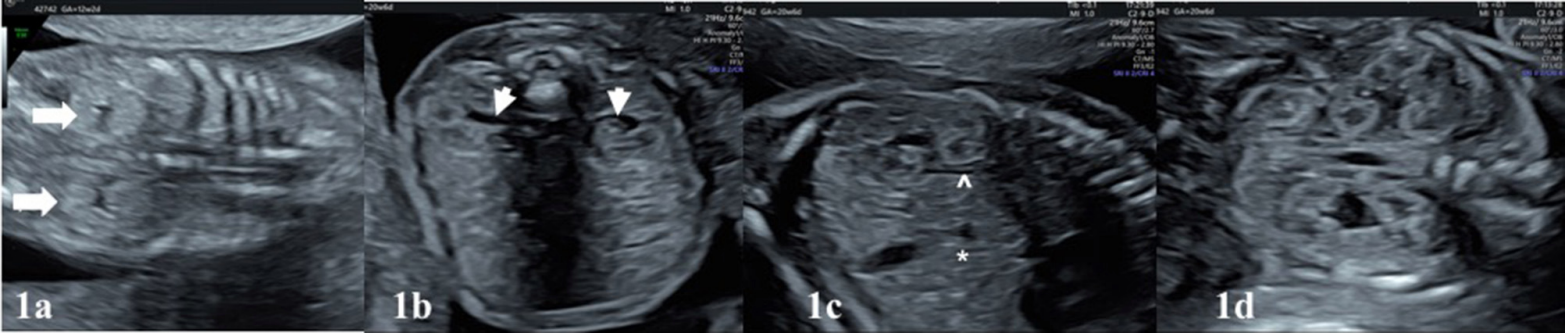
In (a), kidneys of a 12-week fetus. The best visualization of kidneys (white bold arrows) can be obtained in coronal plane with transvaginal ultrasound at this gestational age. (b) Transabdominal ultrasound shows the normal appearance of both kidneys on the axial plane at 20 + 6 weeks of gestation. Kidneys are located both sides of vertebra and renal pelvis (white arrow-heads) oriented toward the midline. (c) Longitudinal plane, echogenicity of the kidney is comparable to the liver (*). Hypoechoic adrenal gland (∧) is located cranial to the kidney. Cortico-medullary differantiation (white chevron) can be noticed. (d) Coronal plane shows two bean-like kidneys in the same section. This plane is useful to compare the kidneys. The corticomedullary differantiation can be noticed easily on the coronal plane.
